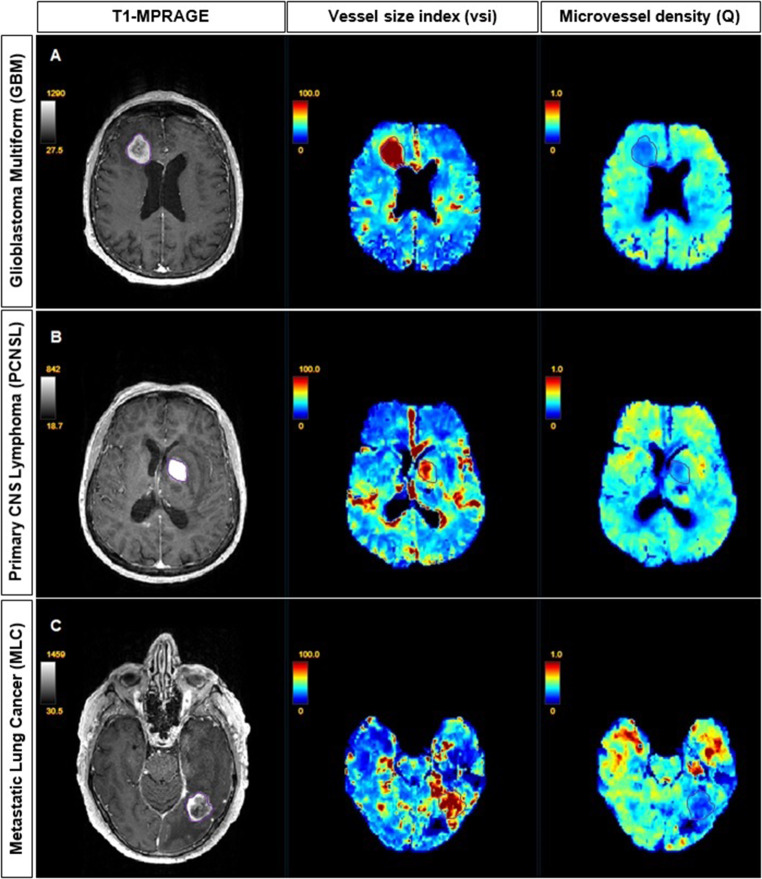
VSI maps shown using the NORA medical imaging platform in patients with a glioblastoma multiforme, b primary CNS lymphoma, c metastatic lung cancer. *Left:* post-contrast T1-weighted images showing enhancement of tumor regions. *Middle:* corresponding mean vessel diameter (vsi) maps. *Right*: corresponding microvessel density (Q) maps. Tumor regions show elevated mean vessel diameters with decreased microvessel densities compared to healthy tissues
